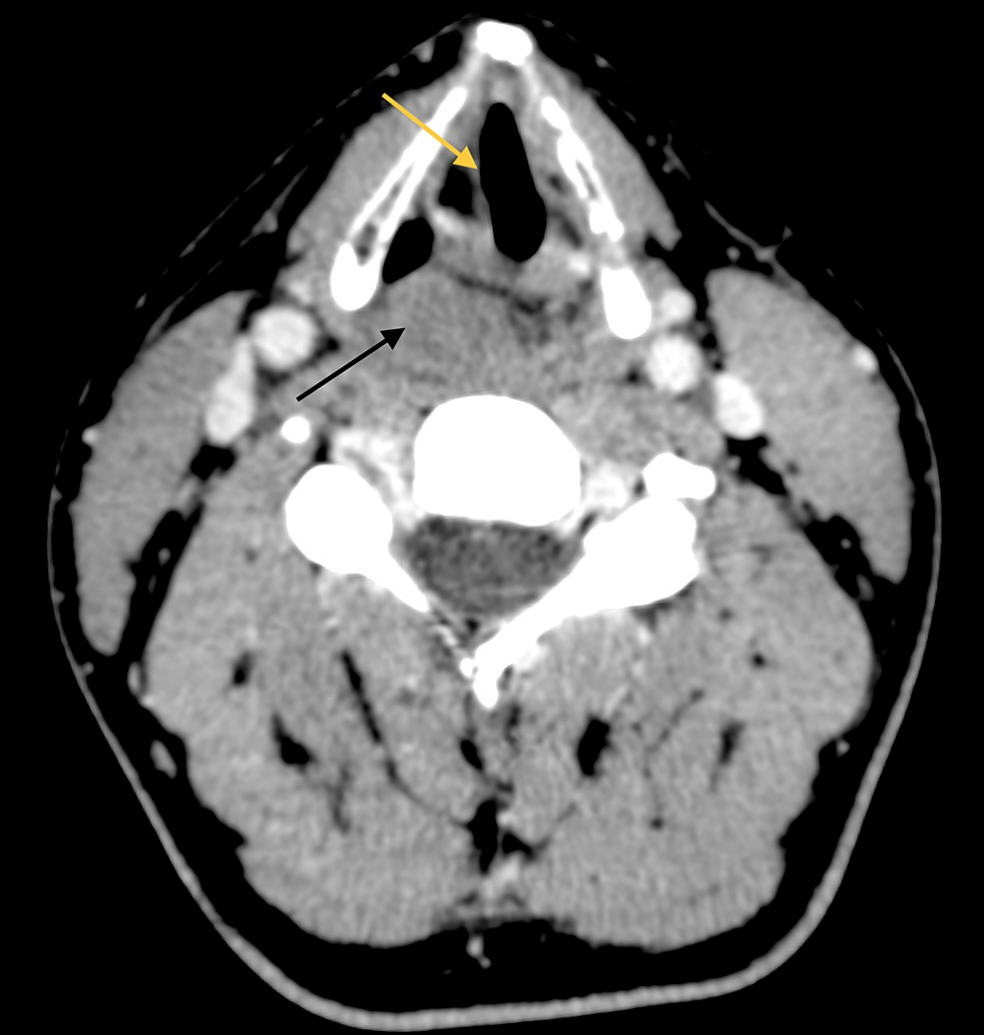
Axial contrast-enhanced computed tomography. Contrast-enhanced CT shows a well-circumscribed solid lesion in the right postcricoid region of the hypopharynx (black arrow). The lesion shows mild homogeneous enhancement. The right vocal cord is slightly medially deviated (yellow arrow).
